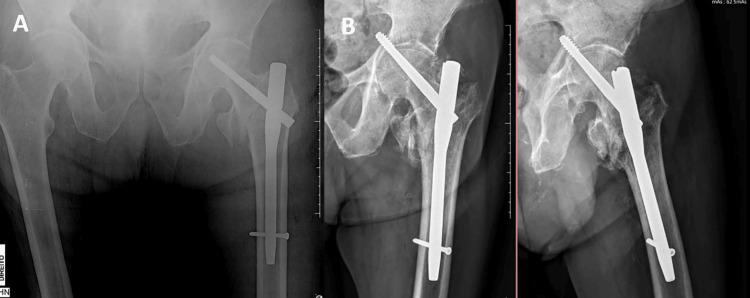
(A) Immediate postoperative radiograph and (B) after 13 weeks, showing cut-through.
